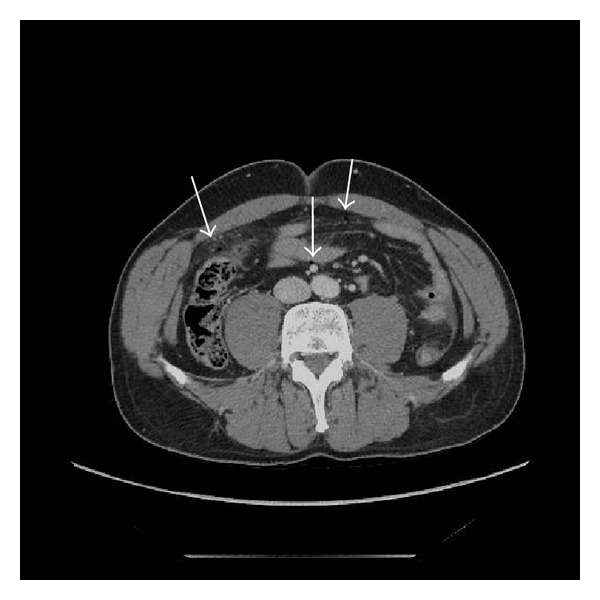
Contrast enhanced axial CT of the abdomen in a 45-year-old male with an inguinal trauma, showing free intraperitoneal air bubbles.
